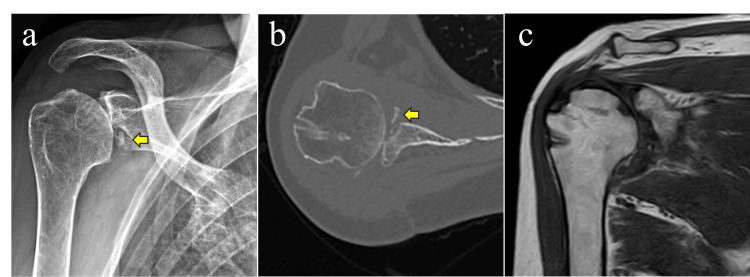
Imaging findings at one year postoperatively (a) Plain radiograph showing nonunion of the glenoid fragment (arrow) with articular incongruity. (b) Axial CT view demonstrating nonunion and fragmentation of the glenoid fragment (arrow). (c) MRI confirming rotator cuff healing.
